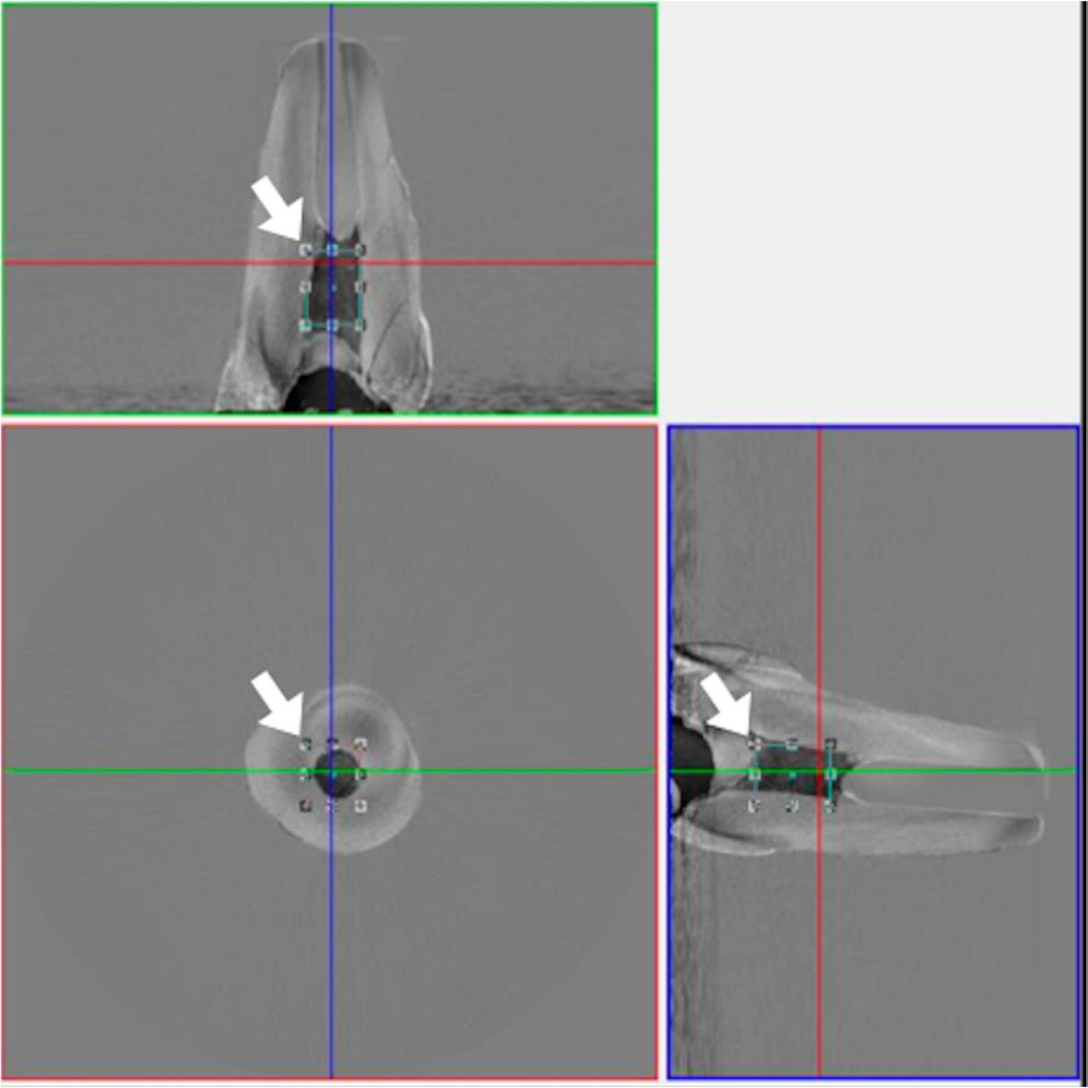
The representative examination of µ-CT data (left) shows the determination of the regions of interest at different planes (white arrows). The representative screenshot of CTAn software (right) shows an image analysis of the overlapped scans (red arrow)
